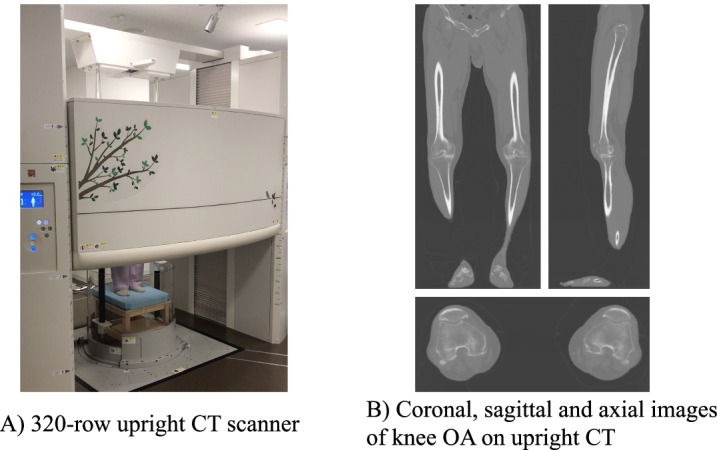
Upright computed tomography (CT) scanner and the acquired images. A A 320-row upright CT scanner (prototype TSX-401R; Canon Medical Systems, Otawara, Japan) was used to acquire images from the hip joint to the end of the foot in the standing position. The approximate acquisition time was 10–20 s. B Acquired images of knee osteoarthritis (OA) with an upright CT scanner. The image qualities of the upright CT scanner range from good to excellent [11]
